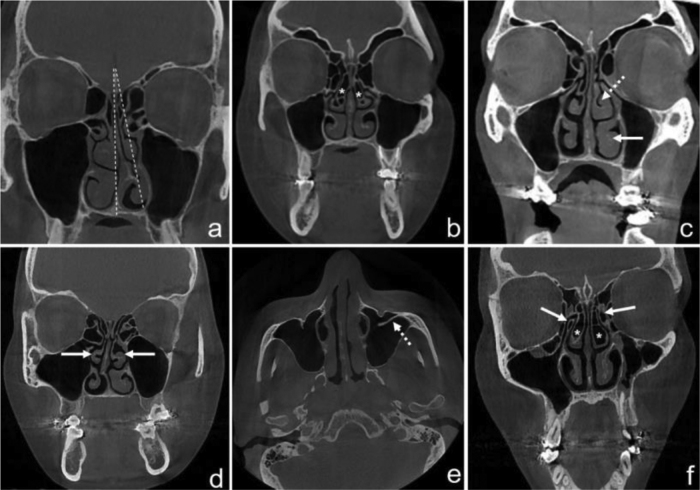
CBCT images of sinonasal anatomical variations. Septal deviation angle (a); bilateral CB (asterisk) (b); middle concha hypertrophy (MCH) (dashed arrow), inferior concha hypertrophy (ICH) (white arrow) (c); bilateral paradoxical middle concha (PMC) (white arrows) (d); presence of septa in the maxillary sinus (dashed arrow) (e); bilateral uncinate process pneumatization (UPP) (white arrows) and CB (asterisk) (f).
